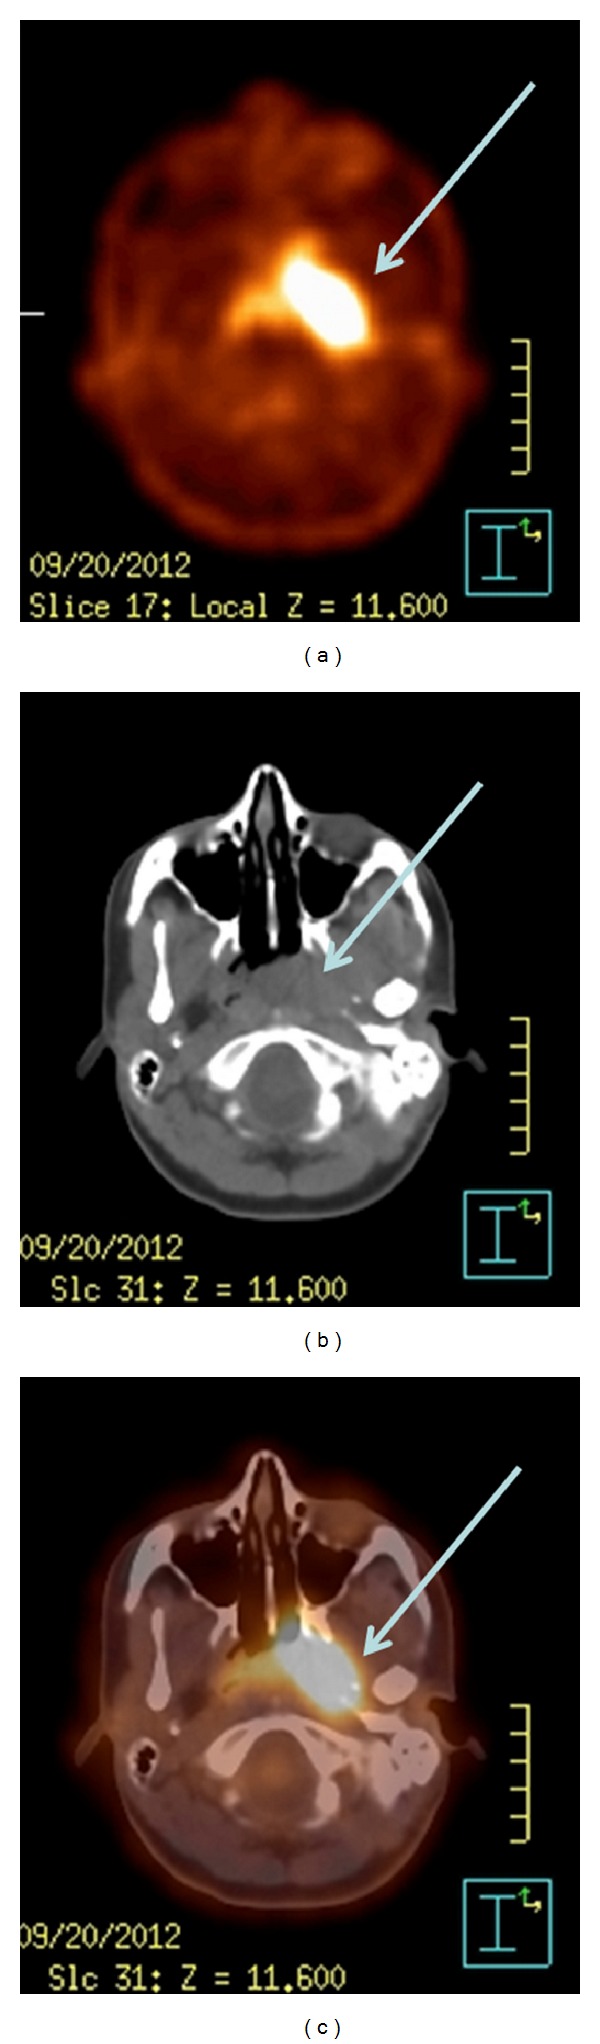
Images showing intense 18F-FDG uptake in rhinopharynx: (a) PET, (b) CT, and (c) PET-CT.
